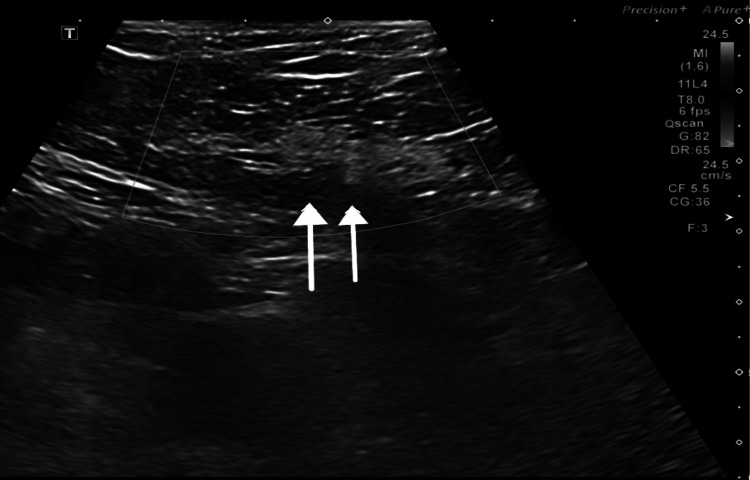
Ultrasound of the abdomen showing (white arrows) oval-shaped hyperechogenic area within the abdominal wall, non-vascular on color Doppler, suggesting fat necrosis or lipoma.
